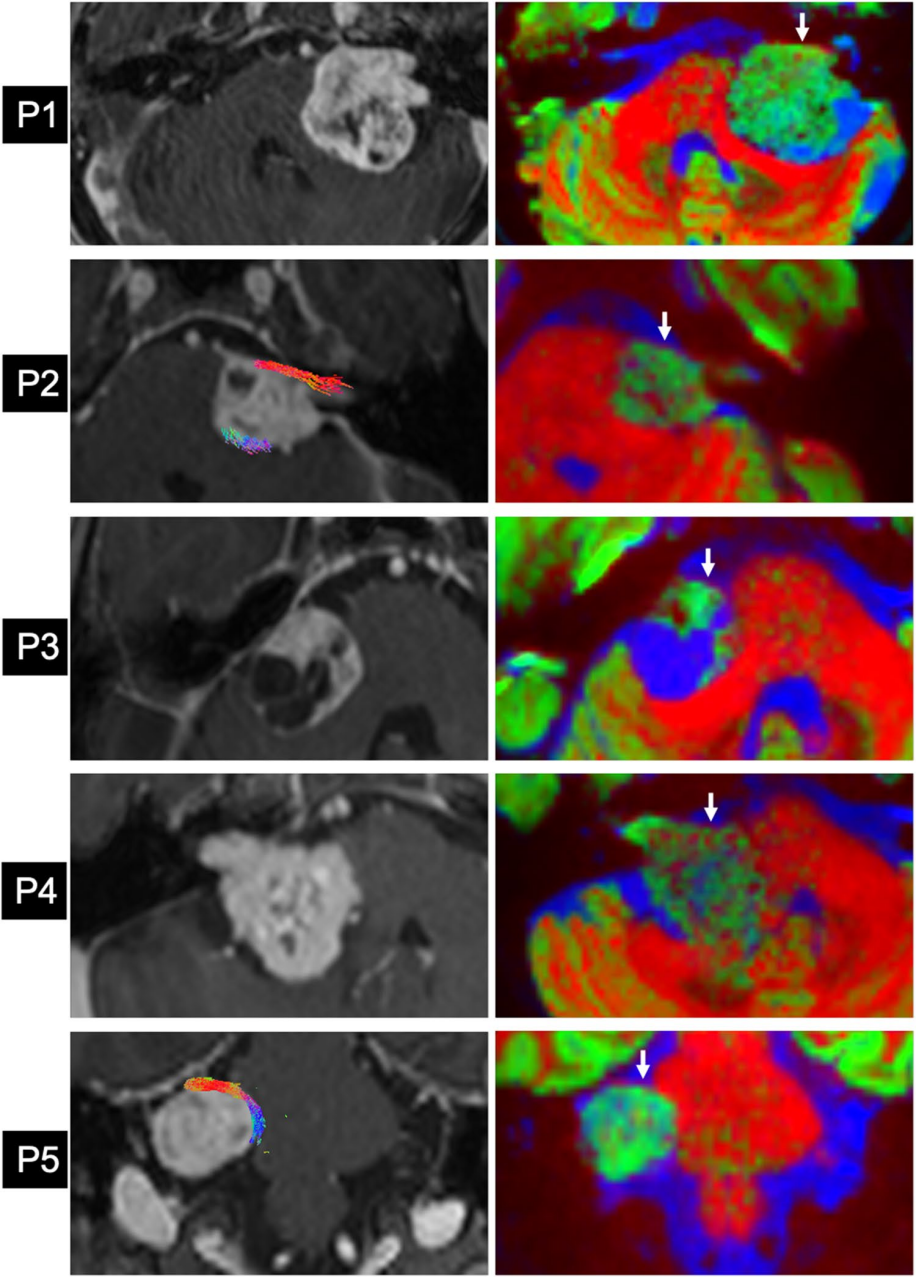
Colour tissue mapping and tractography of the facial nerve in patients with vestibular schwannoma (P1–P5). *Left*: contrast-enhance T1-weighted (ceT1) MRI scan of vestibular schwannoma. Tractography results overlaid on P2 and P5 (tractography not successful in other patients). P1–4: axial ceT1 MRI, P5: coronal ce-T1 MRI. *Right*: corresponding CTM image delineating the facial nerve (arrow). Colour legend: *red*, WM; *green*, GM/tumour; *blue*, CSF/cystic fluid
